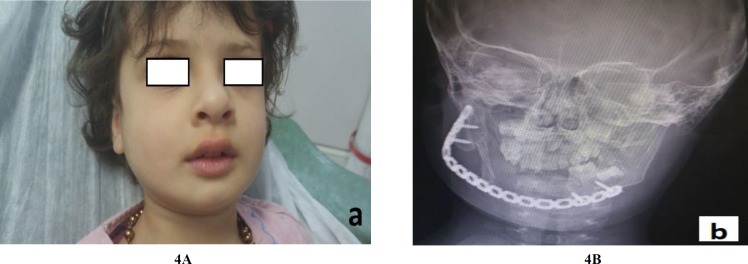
Clinical (A) and radiographic (B) features of case 1 at 6 months after the operation. No residual tumor is present
